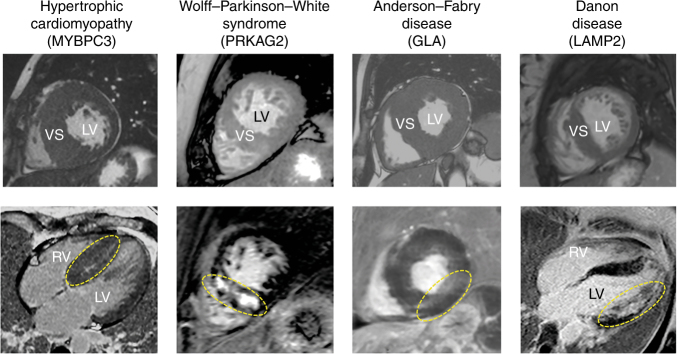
Cardiac magnetic resonance images of example patients with sarcomeric hypertrophic cardiomyopathy and three of its mimics identified in our cohort. Top: cine two-dimensional images in short-axis view using balanced turbo-field echo sequencing. Bottom: late gadolinium enhancement patterns (circled in yellow) in four-chamber (first and last panel) and short-axis view, using spoiled inversion recuperation turbo-field echo three-dimensional sequencing. *LV* left ventricle; *RV* right ventricle; *VS* ventricular septum
